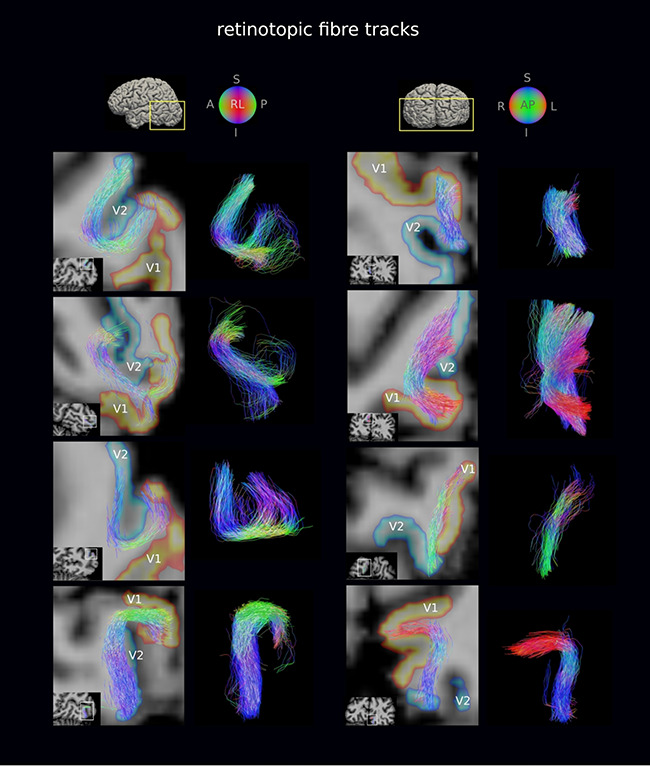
Retinotopic V1–V2 fiber tracks were generally short (16 mm mean length) and followed the pattern of cortical folding. A representative subset of retinotopic V1–V2 fiber tracks are shown for the flexible surface coil DWI of a representative participant. Fiber tracks are shown on oblique slices and are superimposed on the *T*_1_w image transformed to DWI space. The volumetric V1 and V2 retinotopic segments transformed to the DWI space are also shown. The detected retinotopic fiber tracks followed the pattern of cortical folding, but not all were strictly U-shaped. A, L, R, I, S, P: anterior, left, right, inferior, superior, posterior.
